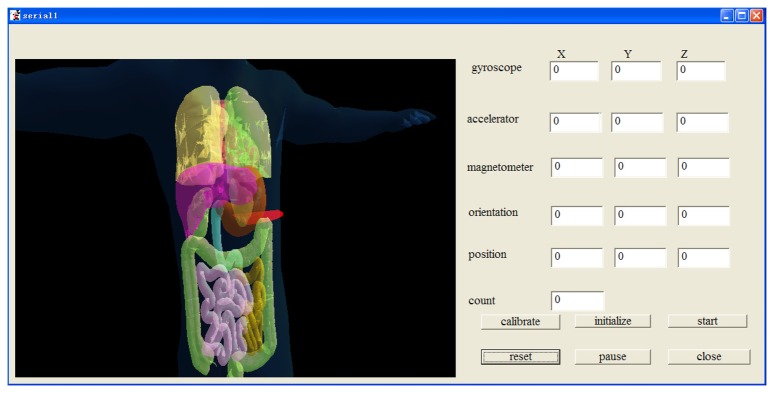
The interface of the ultrasonic endoscope tracking system.
